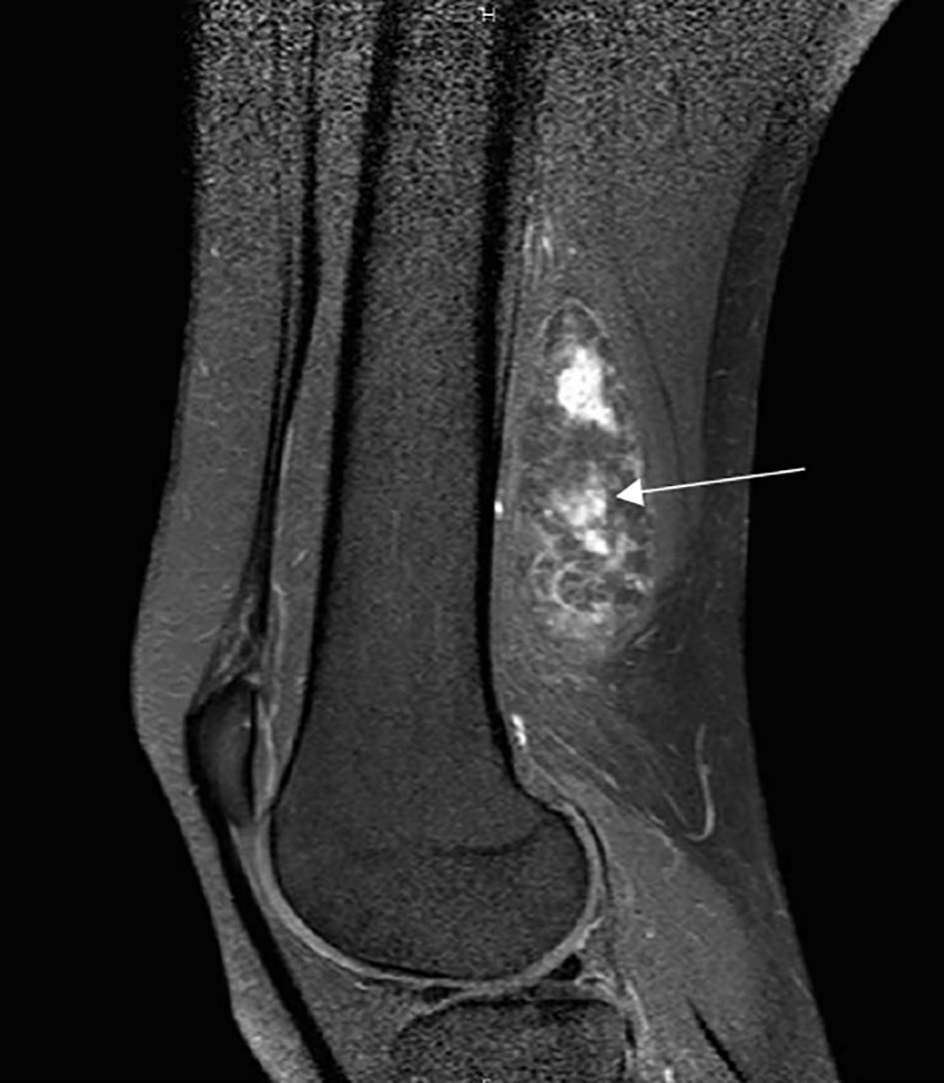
A sagittal T2-weighted MRI shows hyperintense signal in the mass with a heterogeneous aspect.
